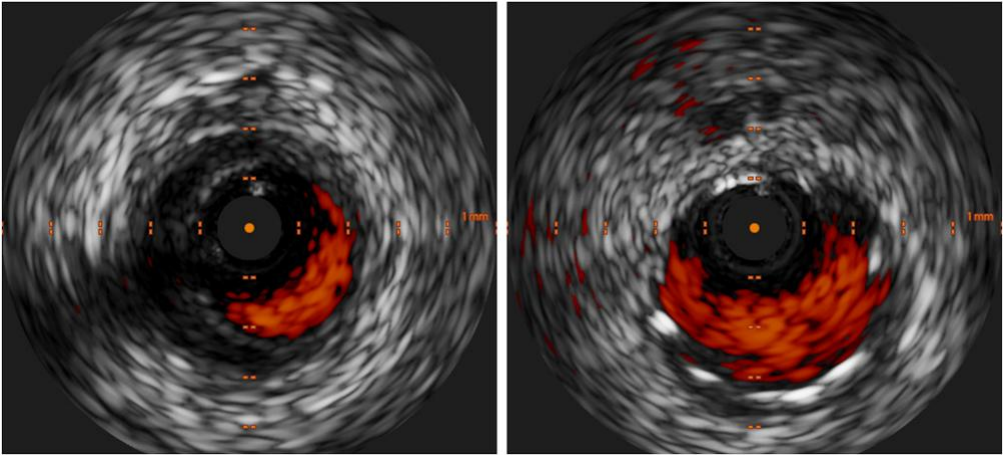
Intravascular ultrasound visualizing acute coronary artery dissection. IVUS diagnosed dissection of LAD (left) and was performed after successful stenting of dissection (right).
